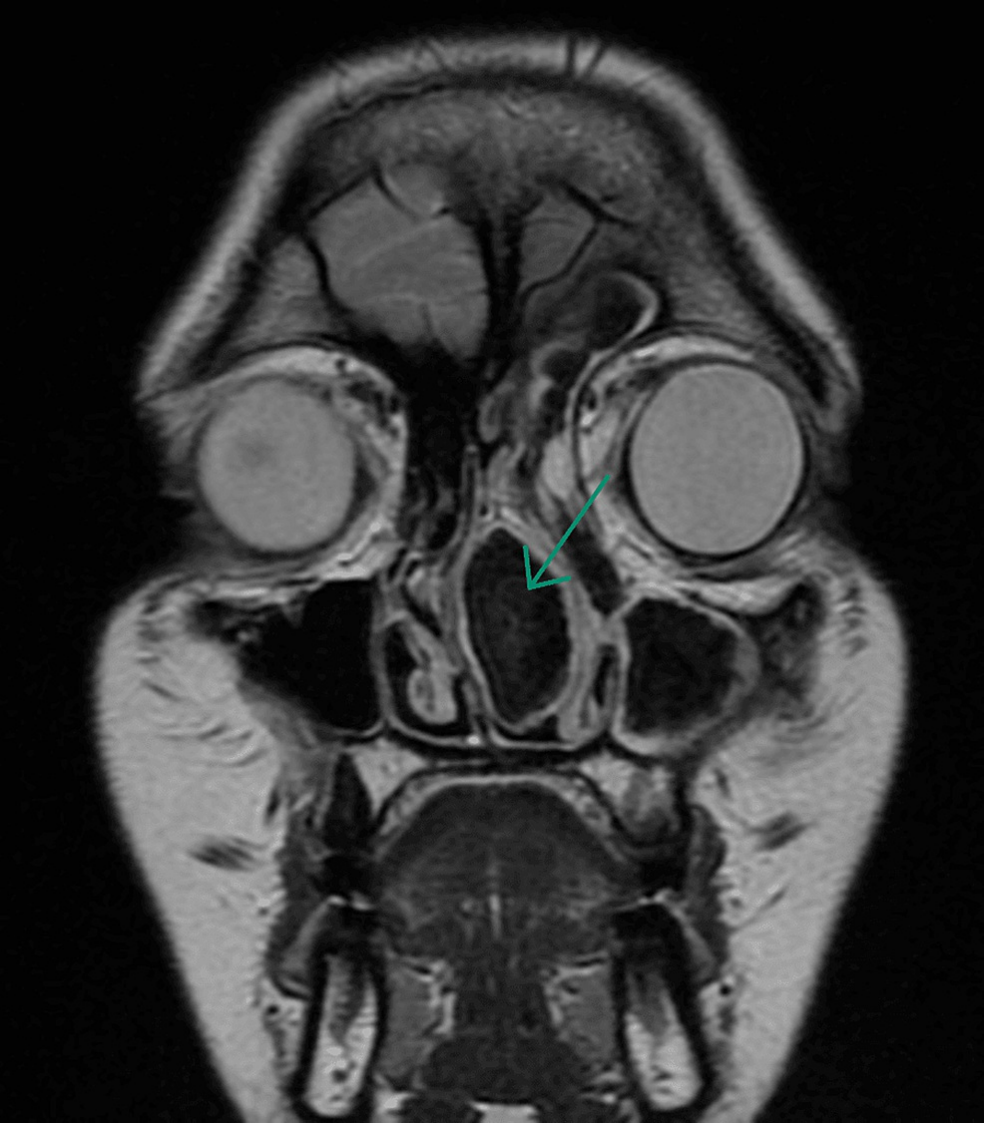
T2-weighted MRI showing the low signal intensity of the mass.
